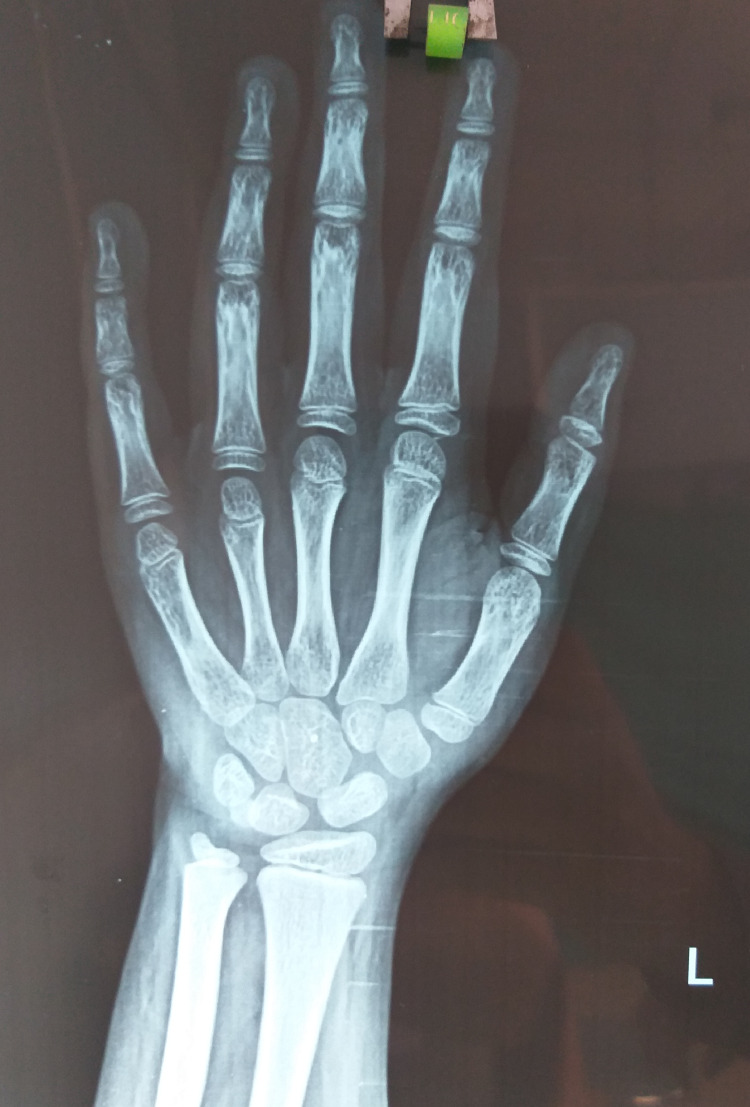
X-ray of the left hand showing advanced bone age.
